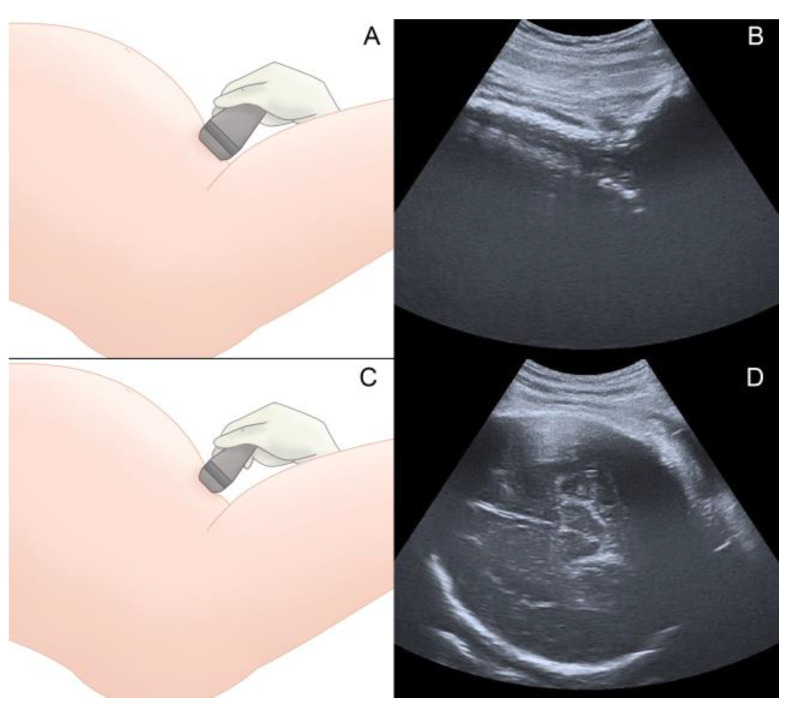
(A) Transabdominal ultrasonography with the probe placed longitudinally and tangentially to the abdomen. (B) Identification of the fetal cervical spine and occipital bone in a sagittal transabdominal plane. (C) Transabdominal ultrasonography with the probe placed transversely at the suprapubic region. (D) Visualization of the brain structures in a transverse transabdominal plane.
